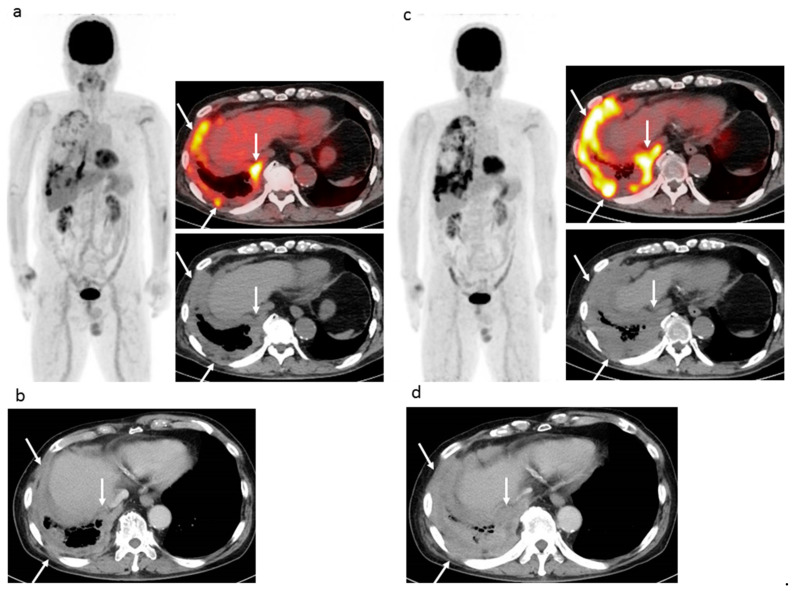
74 year-old man with right epithelioid malignant pleural mesothelioma (cT2N0M0), who previously received six cycles of first-line chemotherapy (pemetrexed + cisplatin) and then 12 cycles of nivolumab as second-line chemotherapy. (a) Pre-nivolumab treatment FDG-PET/CT shows multiple areas of strong FDG uptake in areas of right pleural lesions (arrows). (b) Pre-nivolumab treatment contrast-enhanced CT shows mass-forming thickness of right pleura (arrows). (c) Post-treatment FDG-PET/CT after 12 cycles of nivolumab shows remarkable progression of multiple pleural lesions (arrows) and appearance of new pleural lesions. (d) Post-treatment contrast-enhanced CT after 12 cycles of nivolumab shows remarkable progression of pleural lesions (arrows). EORTC, PERCIST, imPERCIST, and CT criteria (modified RECIST and RECIST 1.1) indicated PMD or PD due to remarkable progression and appearance of new lesions. In FDG-PET/CT results, the SULpeak sum of the five highest level pleural lesions was increased by 98.6%. In CT findings, the sum size of six pleural lesions perpendicular to the chest wall was increased by 40.3%. According to the second (c) FDG-PET/CT and (d) contrast-enhanced CT result, the patient started another chemotherapy series (irinotecan + gemcitabine), though was alive at 13.9 months after initiation of nivolumab.
